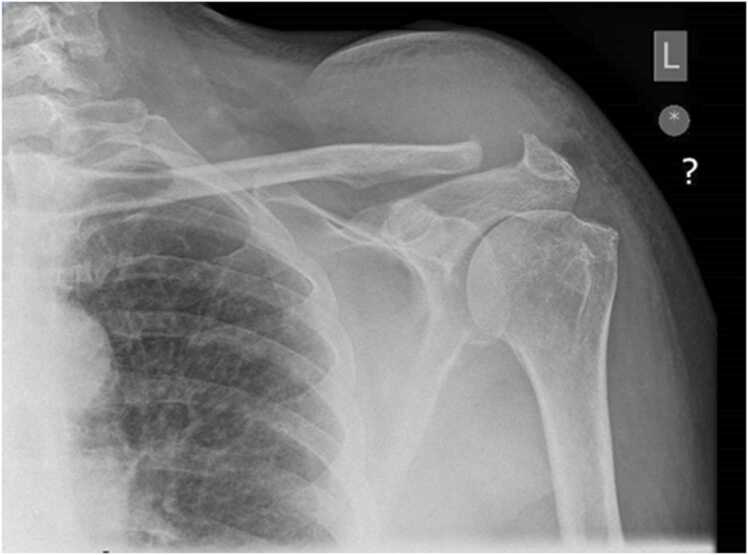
A plain radiograph of the shoulder showed joint space widening with acromioclavicular joint osteoarthritis.
